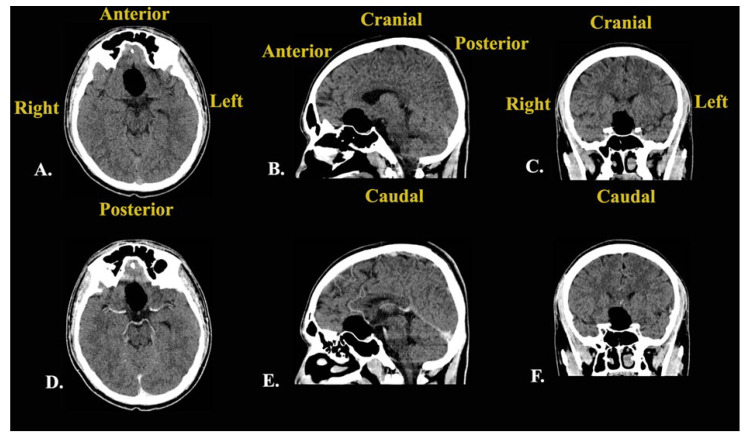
Preoperative brain CT scan: (A) native axial sequence; (B) native sagittal sequence; (C) native coronal sequence; (D) contrast-enhanced axial sequence; (E) contrast-enhanced sagittal sequence; (F) contrast-enhanced coronal sequence.
